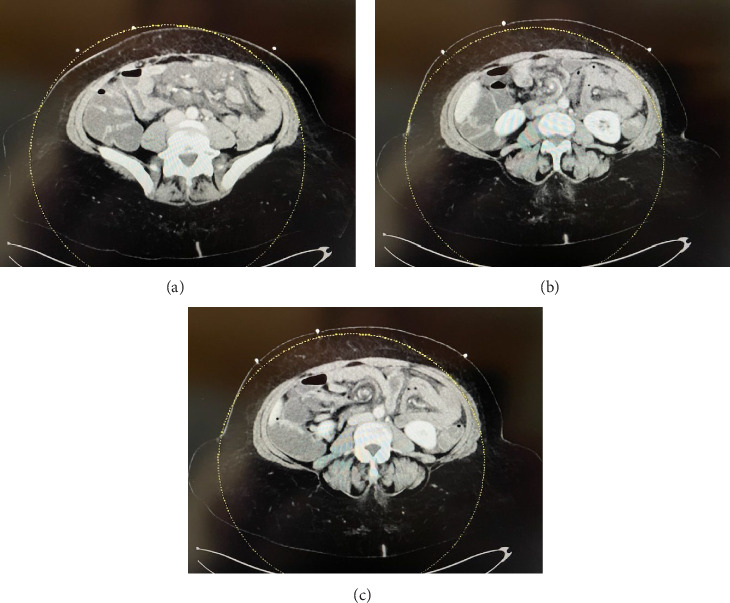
Preoperative CT scan. (a) Pelvis, (b) lower abdomen, and (c) mid abdomen.
